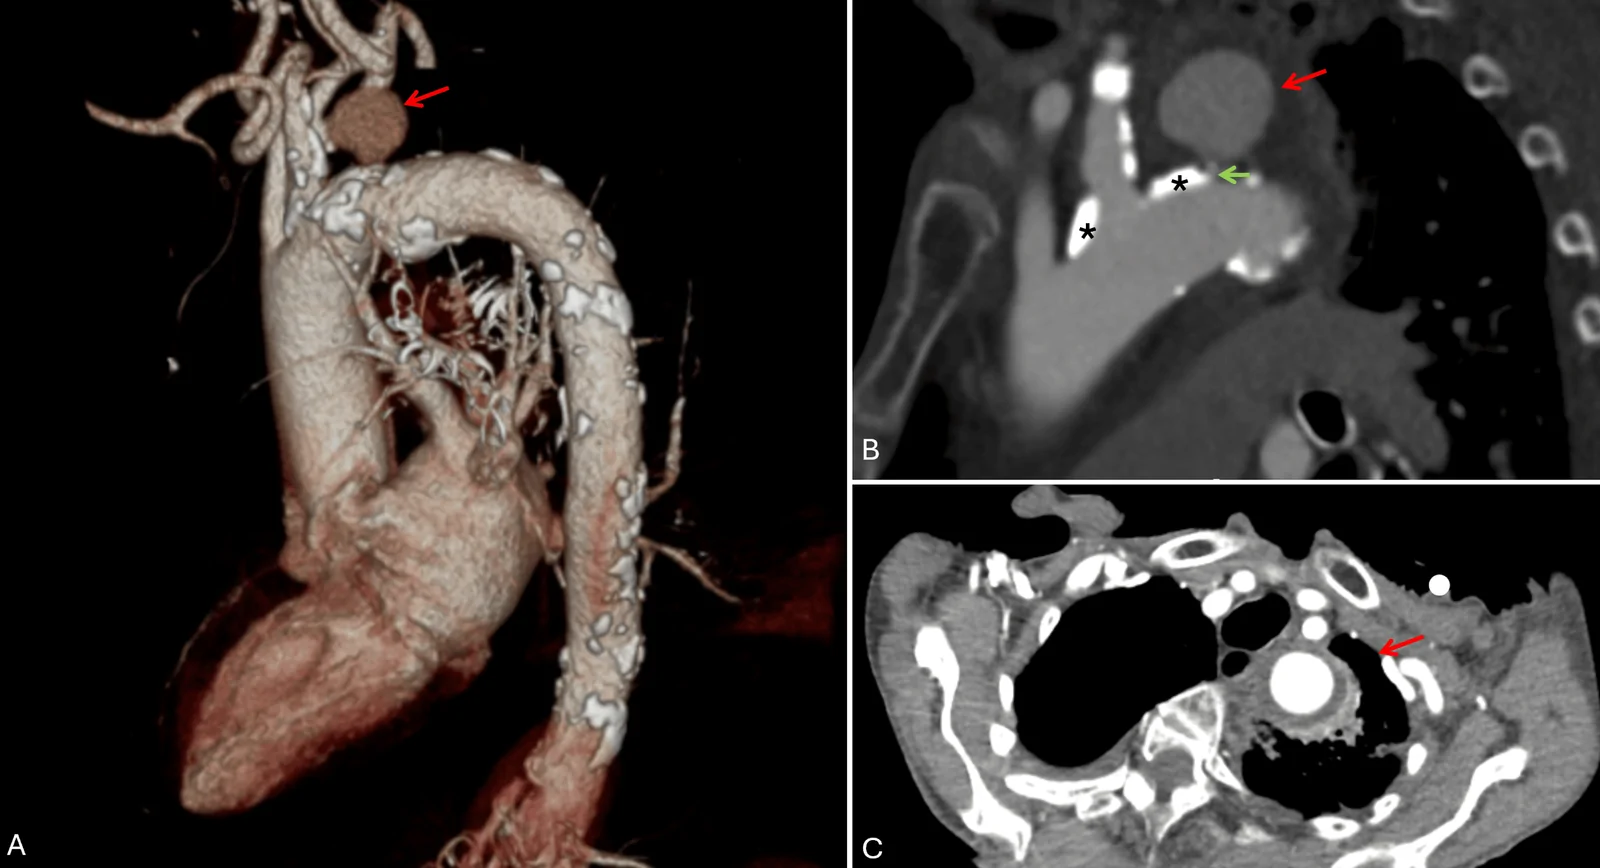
Chest CTA images obtained using an aortic protocol. (A) Three-dimensional reconstruction. (B) Sagittal reformatted image. (C) Axial mediastinal window A saccular outpouching arising from the aortic arch (red arrow) demonstrates the same contrast enhancement as the aortic lumen, consistent with a pseudoaneurysm secondary to a penetrating aortic ulcer. The neck of the pseudoaneurysm is indicated by the green arrow, and adjacent calcified atherosclerotic plaques are marked with asterisks (*). No contrast extravasation into the pleural cavity is identified. CTA: computed tomography angiography.
